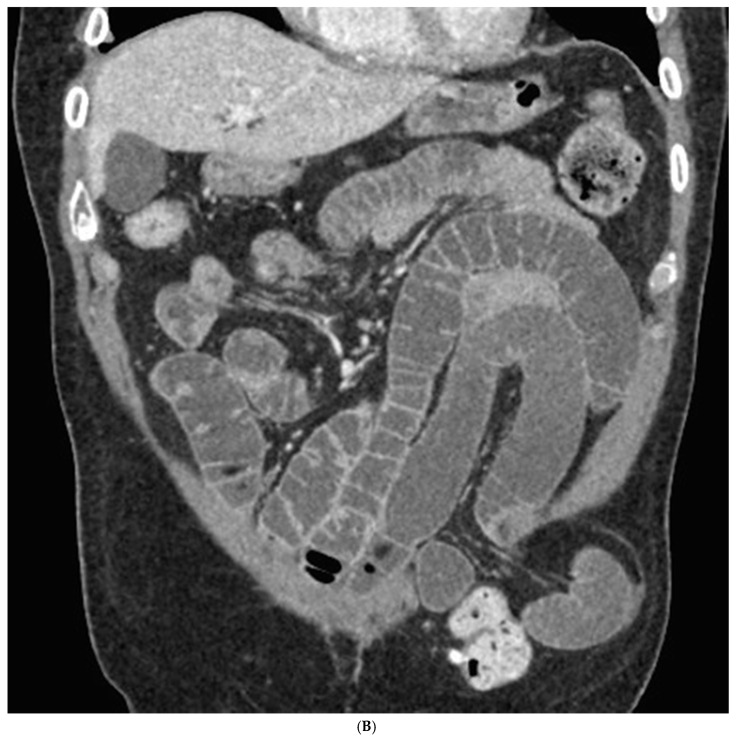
Simple SBO. (A) Dilated and fluid-filled bowel loops with decreased peristalsis; valvulae conniventes are clearly visible but not thickened. No free fluid was detected in the abdominal cavity or between bowel loops. (B) CT with IV contrast, coronal MPR, confirmed a simple SBO diagnosis in caused by a left spigelian hernia.
